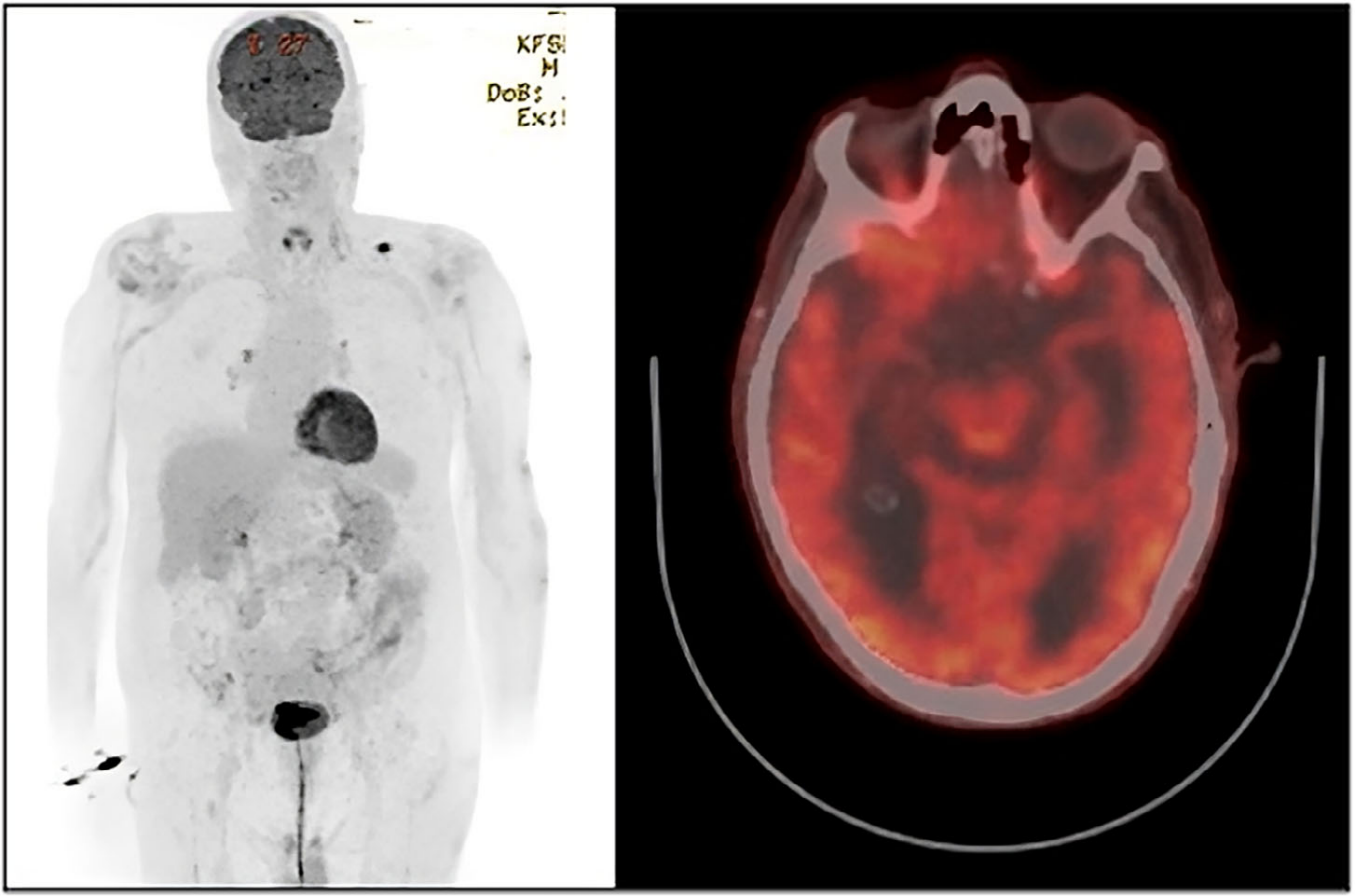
Positron emission tomography (PET) 3 months after commencement of acalabrutinib. This shows complete resolution of intracranial changes and almost complete resolution of fluorodeoxyglucose (FDG)‐avid lymphadenopathy.
